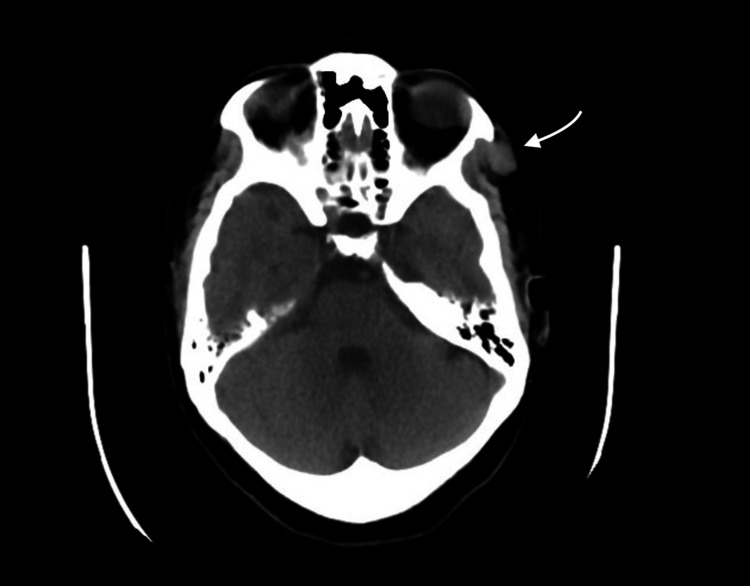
Computed Tomography Scan, axial view The arrow shows the identified lesion
